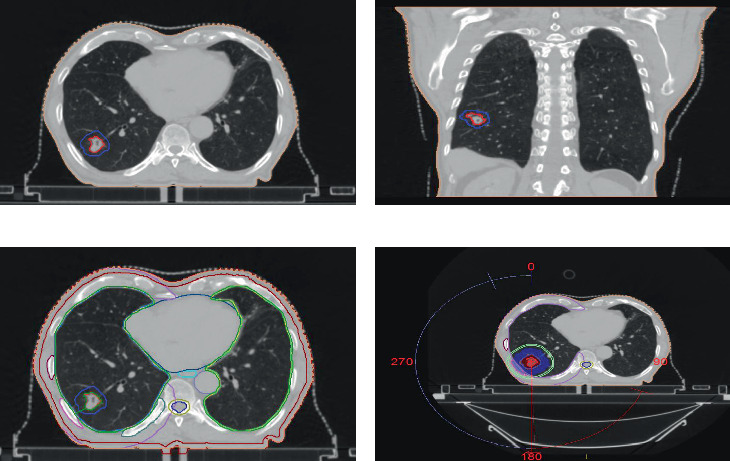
Contouring volumes on computed tomography scan for lung lesion. (a) Axial section: GTV in bright red: 34 Gy, PTV in dark blue: 34 Gy. (b) Coronal section: GTV and PTV. (c) Axial section: skin: maroon, right eight rib: dark red, right ninth rib: pink, chest wall: light purple, heart: green, oesophagus: cyan, spinal cord: dark blue, spinal cord PRV: muddy green, ipsilateral lung: green, contralateral lung: light green. (d) Axial section showing dose coverage of the GTV, dose wash within 2 cm ring.
